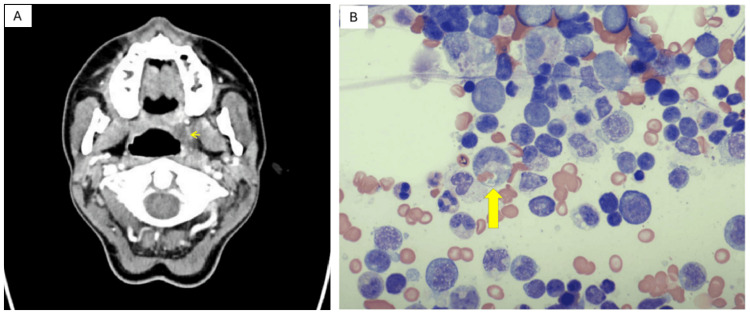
(A) Contrast-enhanced CT scan of the neck demonstrates findings consistent with acute tonsillitis and a dominant left peritonsillar abscess (yellow arrow) measuring up to 1.7 cm in diameter. (B) Bone marrow aspirate smear demonstrates hemophagocytosis, a hallmark of hemophagocytic lymphohistiocytosis (HLH). The image shows activated histiocytes with abundant cytoplasm engulfing hematopoietic elements (yellow arrow).
